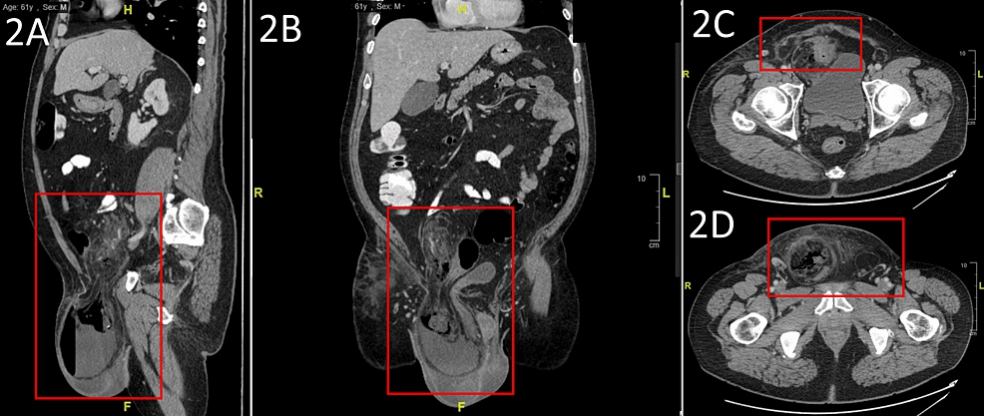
CT-imaging of a large, indurated right-sided inguinal hernia (A) Multiple loops of small bowel in the hernia-sac, possibly sigmoid colon; (B) Imaging also demonstrates possible herniation of right scrotal tissue; (C & D) Significant fat-stranding of adjacent soft tissues.
